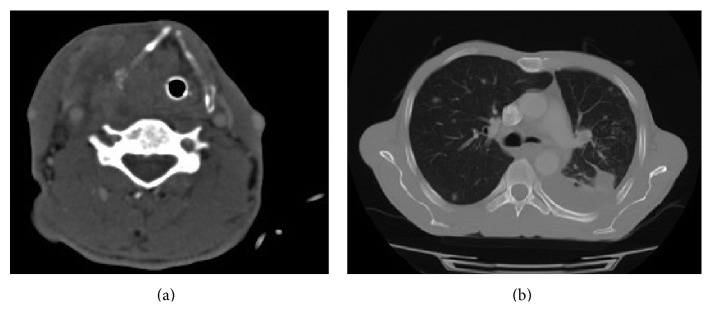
(a) CT scan with contrast enhancement showing a tumoral mass of the right pyriform sinus extending through the extralaryngeal region with thyroid cartilage destruction and prevertebral area involvement. (b) CT scan demonstrating multiple metastases in both lungs.
